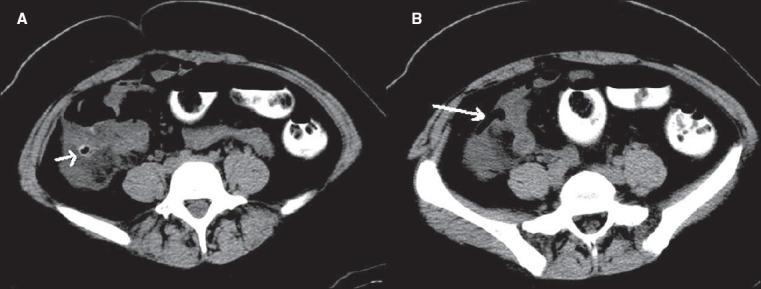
Cecal diverticulitis. Plain CT scan with oral contrast shows an inflamed right-sided cecal diverticulum (short arrow in A) with surrounding fat stranding and an air-filled normal appendix (long arrow in B)
